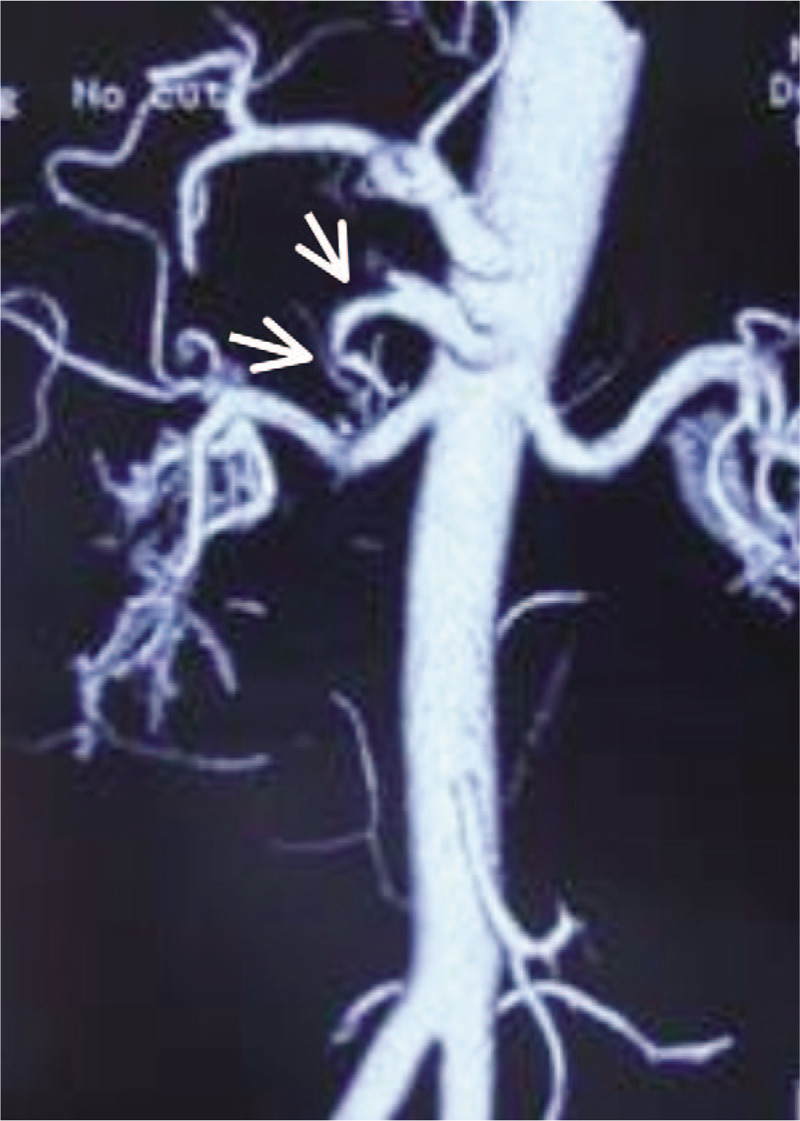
Before the operation, computed tomography angiography (CTA) revealed that superior mesenteric artery (SMA) had occluded completely (white arrow).
